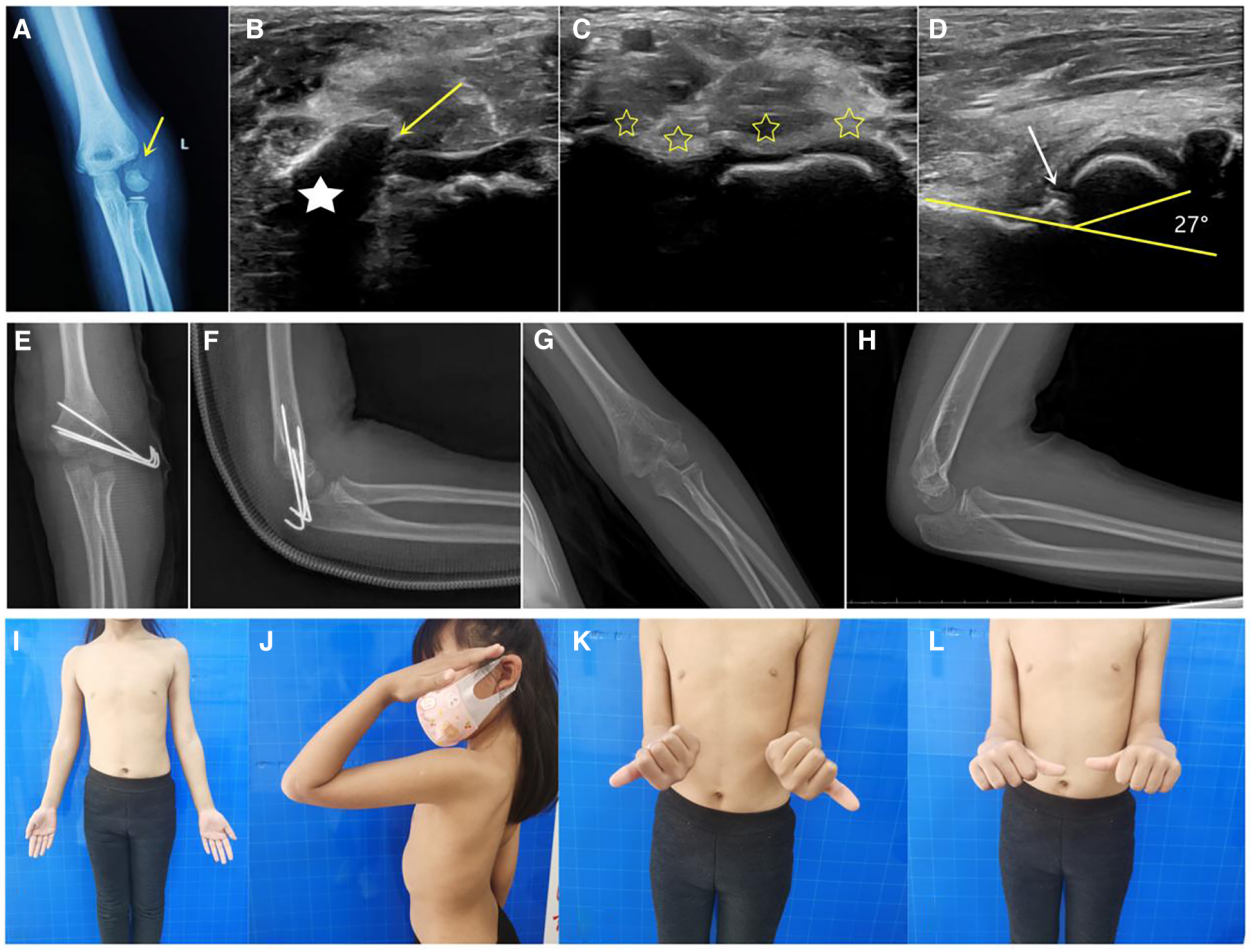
Case of a 6-year-old patient. (A) Radiograph showing the rotated and displaced left humeral lateral condylar fracture. (B) On ultrasonography, the humeral lateral condyle fracture was shown to have rotated, with the asterisk indicating the rotated fragment and the arrow indicating the fracture cartilage line. (C) Via ultrasound-guided closed reduction, the articular cartilage on the coronal plane was visible continuously (asterisk). (D) In the sagittal plane, the normal condylar shaft angle was 27°, without steps or displacement. (E,F) Immediate postoperative radiograph showing good fracture alignment. (G,H) Radiograph at the 19-month follow-up after surgery showing fracture healing and no capitellum necrosis. (I–L) At 19 months after surgery, the patient had good elbow function and no scarring.
